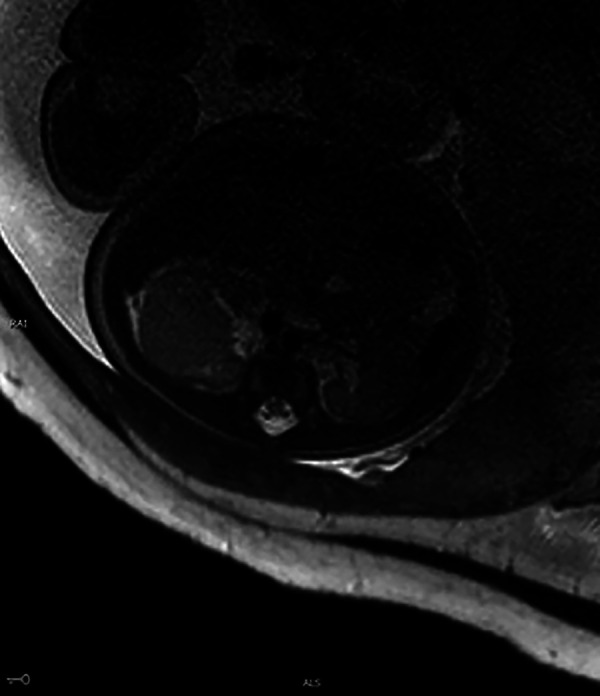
MRI of the foetus. A large, irregular mass (28 mm × 25 mm × 21 mm) was noted with isointense on T2WI.
